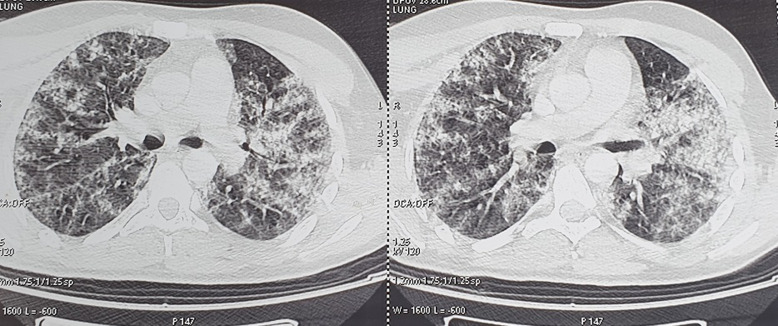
findings on chest computed tomography (CT) on admission showing multiple frosted glass areas and central and peripheral consolidation diffusely distributed over the two lungs
